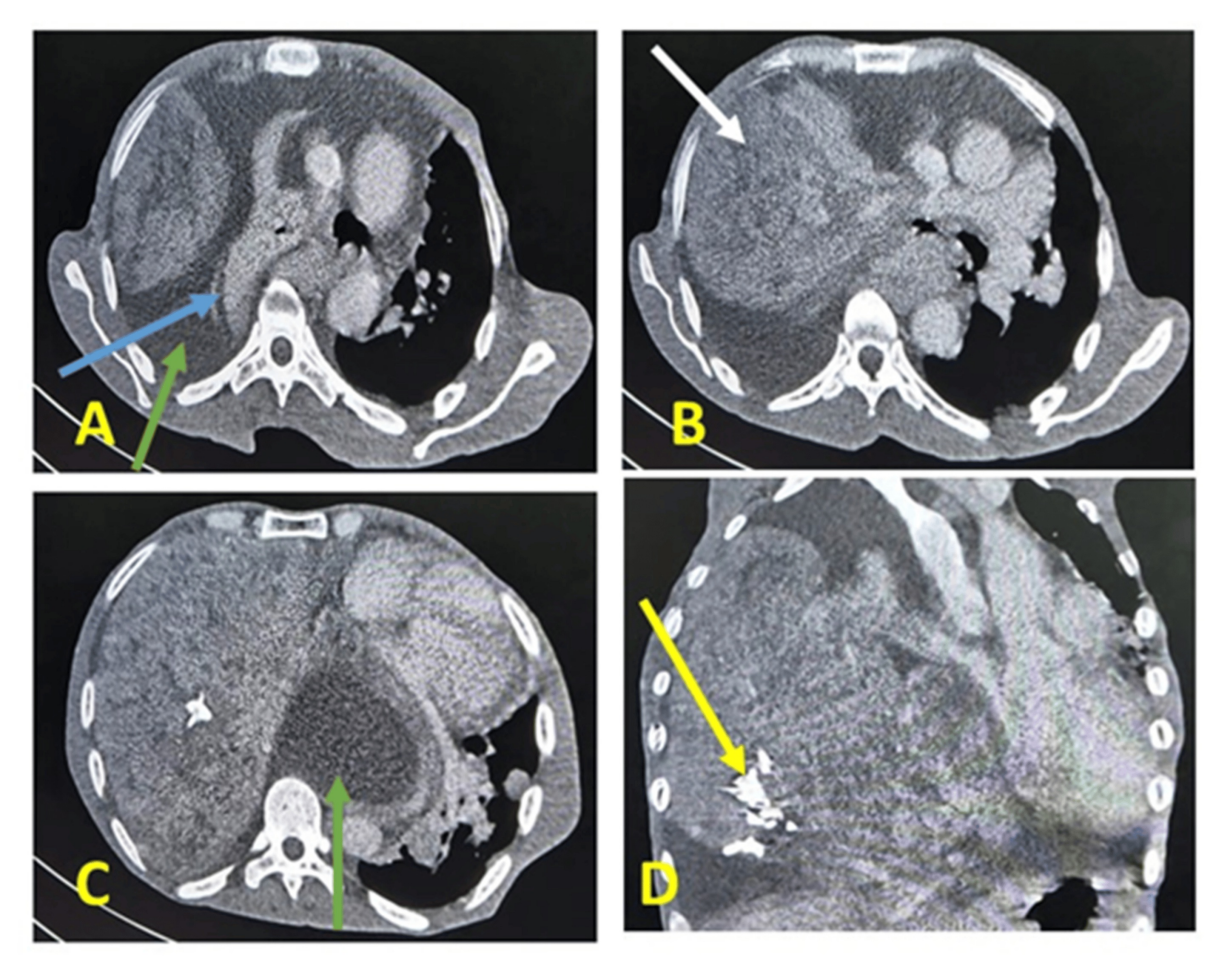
Chest computed tomography after biopsy (A-D) Reveal a worsening pleural effusion (green arrow) around the tumor (white arrow), with calcifications (yellow arrow), left lung atelectasis (blue arrow), and a shift in the mediastinum.
